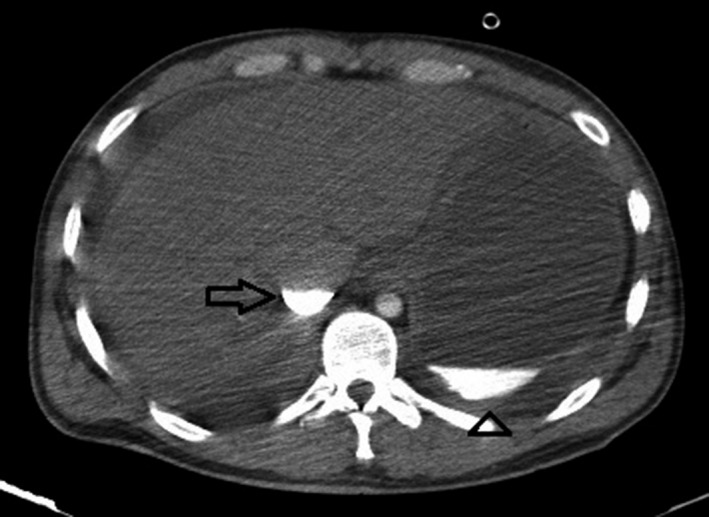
Contrast‐enhanced MDCT angiography shows radiopaque substance in the stomach despite no use of oral contrast (arrowhead). Early enhancement of the IVC (inferior vena cava) during arterial phase of the CT study with contrast‐fluid level formation (arrow) is evident.
